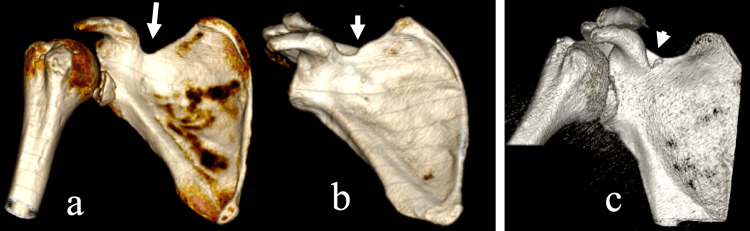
Common morphological types of the suprascapular notch as seen on 3D-CT models. Wide depression of the superior border forms of the (a) scapula (long arrow), (b) U-type (short arrow), and (c) V-type (arrowhead).
